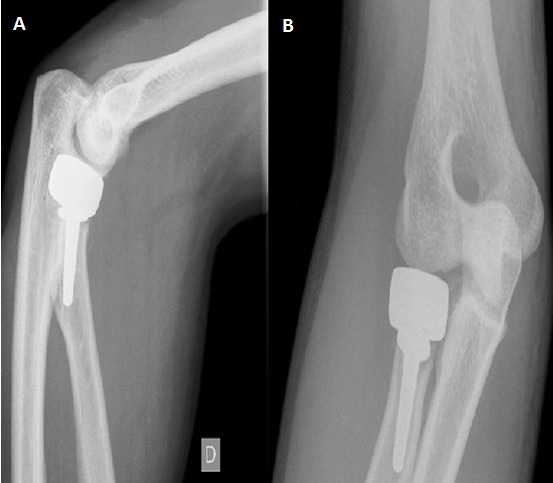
Radiographie du coude droit de face et de profil: arthroplastie de la tête radiale (A,B)
